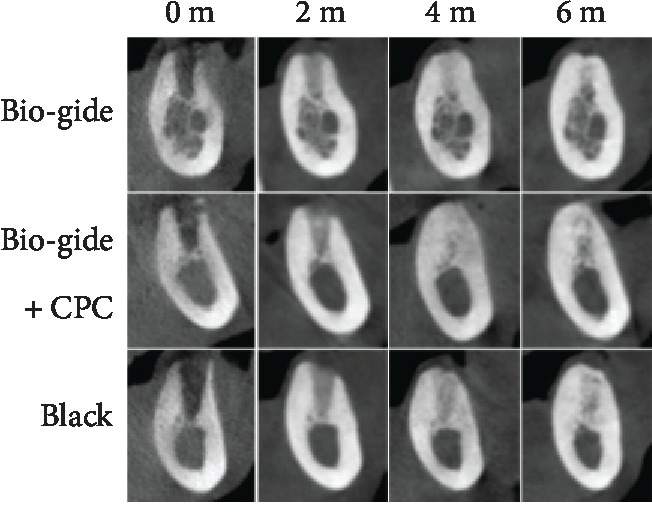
Representative CBCT images of extraction sockets at several time points after surgery.
